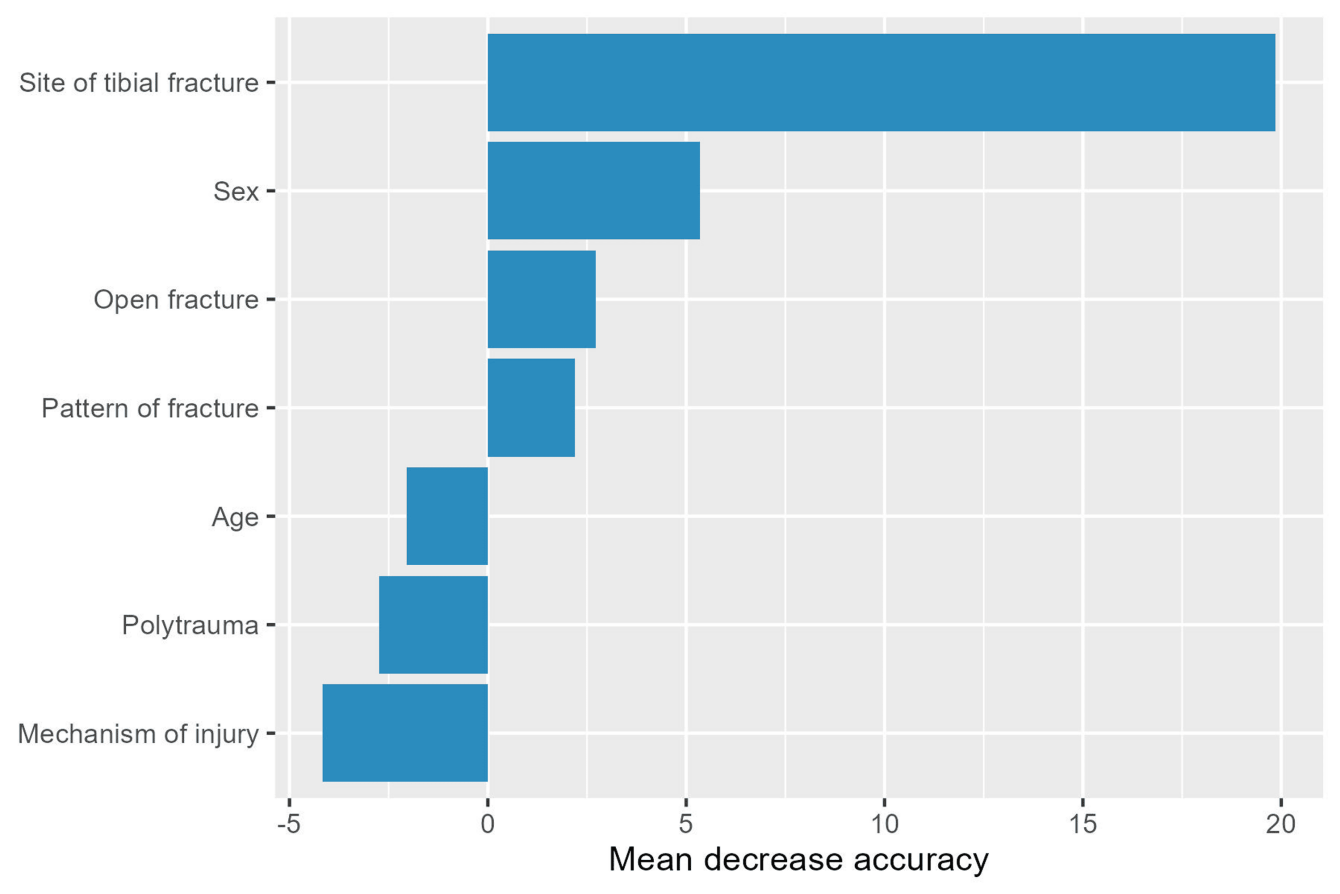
Random forest variable importance for predicting missed fragments on radiography
